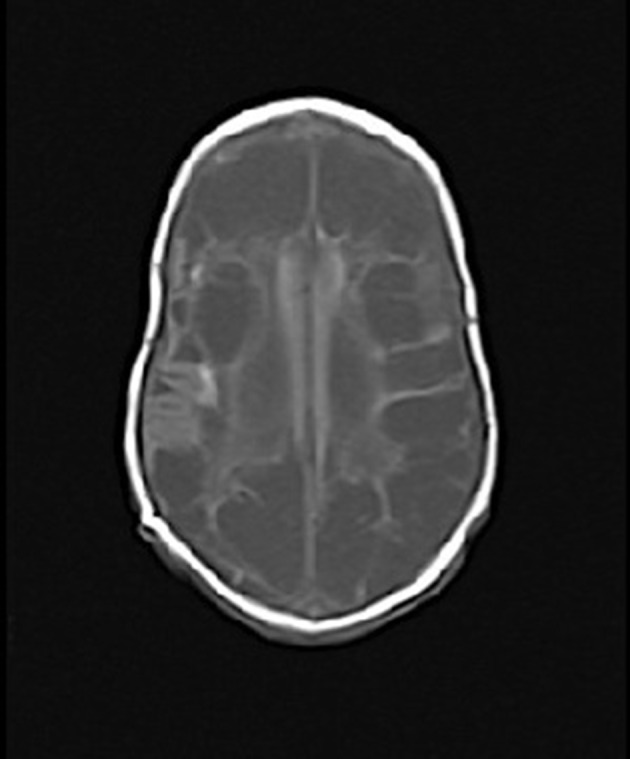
MRI of brain of infant 3 weeks post-second episode of GBS sepsis.
